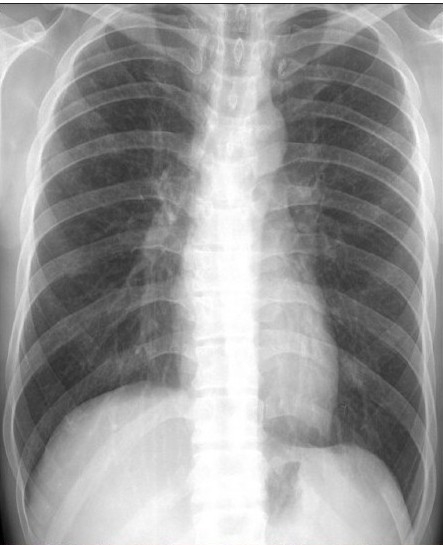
Posteroanterior radiograph of the chest shows fine reticular opacities in the upper and middle zones of both lung fields.
